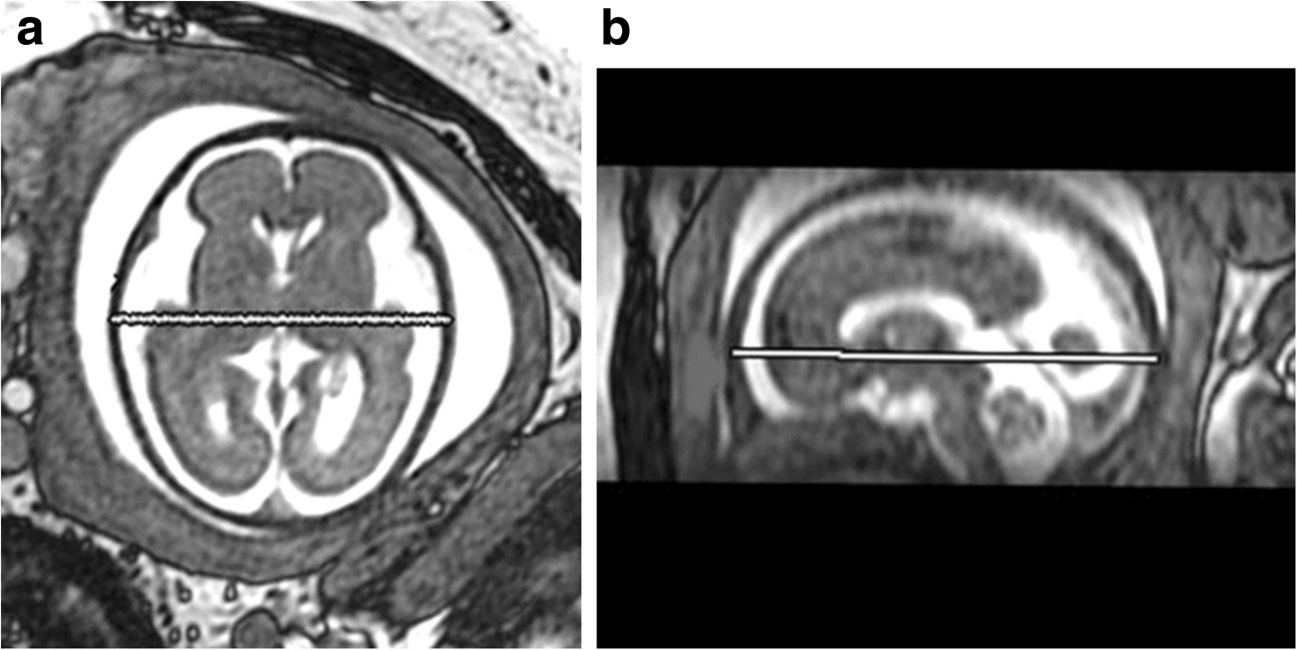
Axial and Sagittal MR images from 3D datasets showing the measurement of bi-parietal diameter (a) and occipito-frontal diameter (b)
